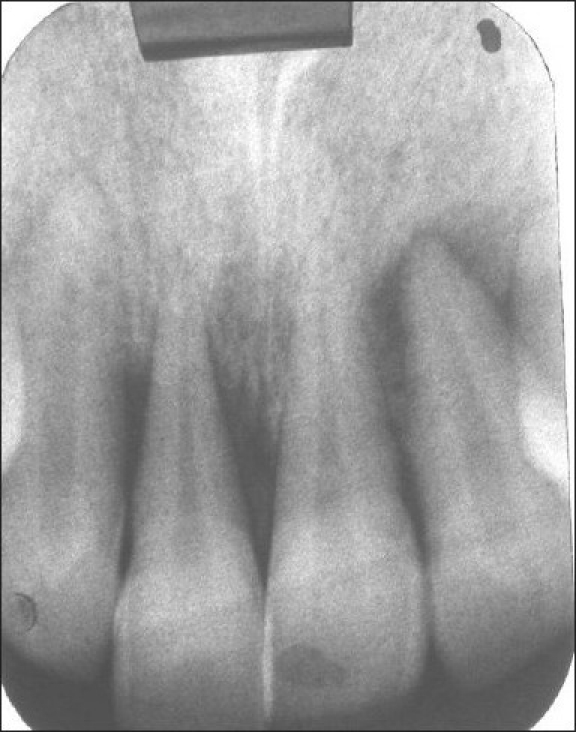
Pre-treatment radiograph showing osseous defect
